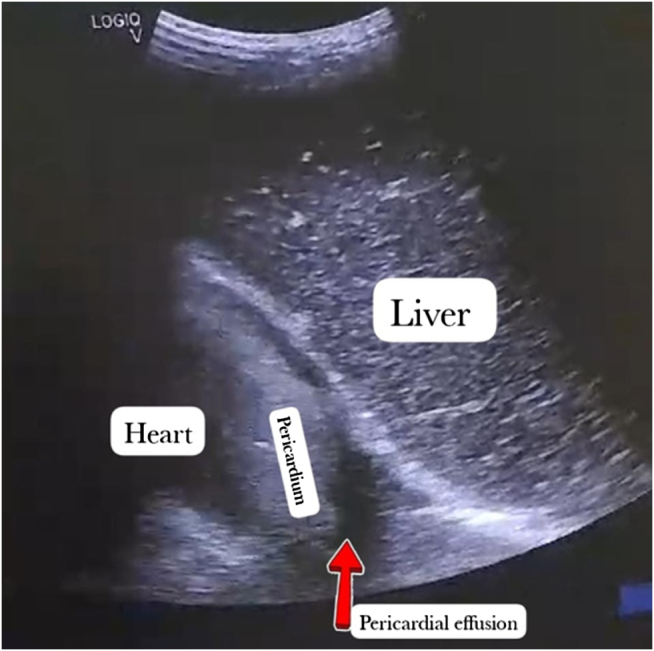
Ultrasonography showing pericardial effusion.
